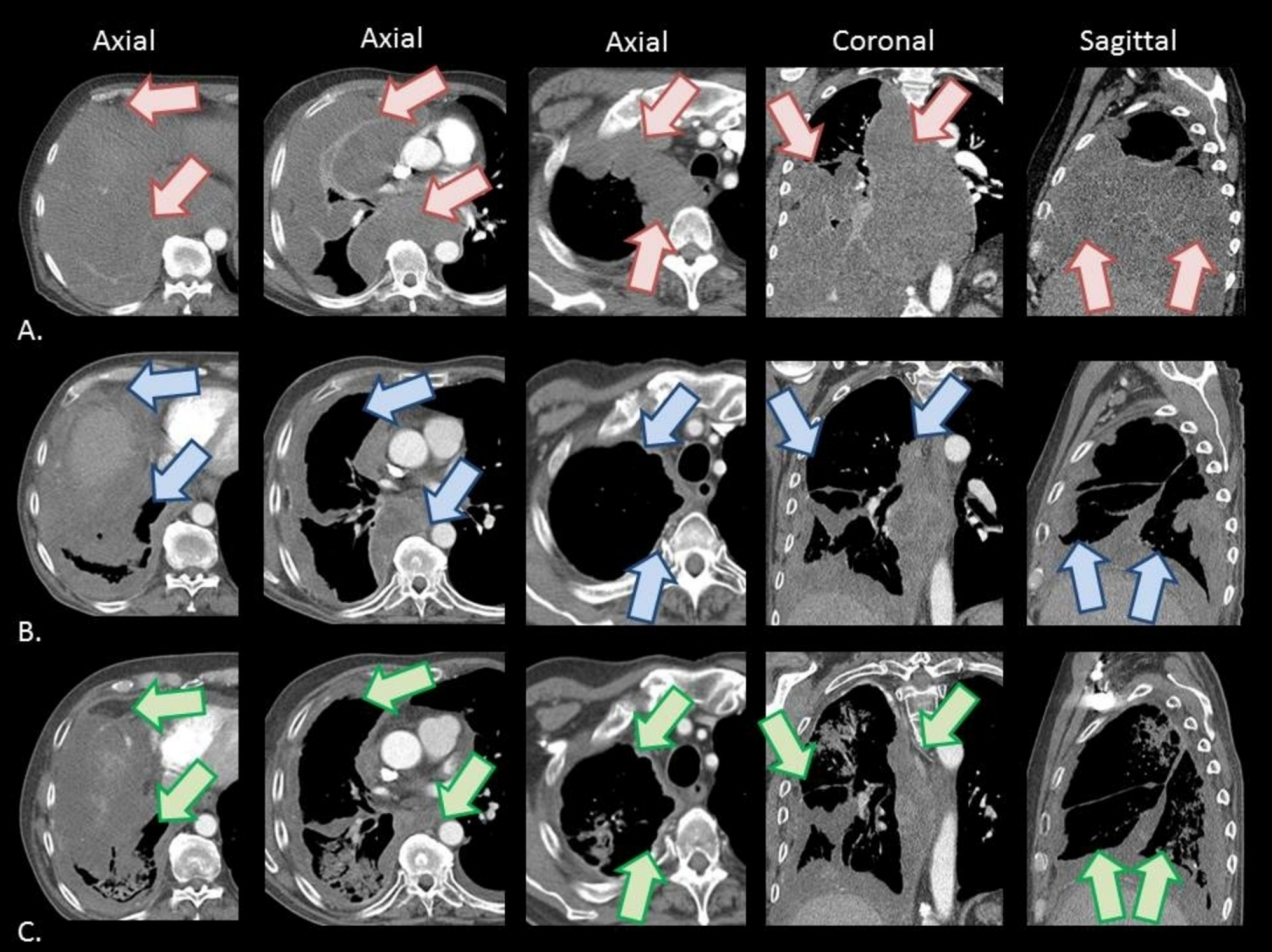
Pre and post-treatment chest CT scans Axial (from left to right, at the levels of the diaphragm, great vessels, and inferior clavicular heads), coronal, and sagittal IV contrast-enhanced CT images obtained (A) prior to immuno-gene therapy, and at (B) three months and (C) six months following immuno-gene therapy, revealing a sustained dramatic decrease in burden of pleural tumor. Representative regions of the tumor prior to immuno-gene therapy are indicated by red arrows, with representative regions of tumor response to therapy at three months and six months following immuno-gene therapy indicated by blue arrows and green arrows, respectively. CT, computed tomography; IV, intravenous
